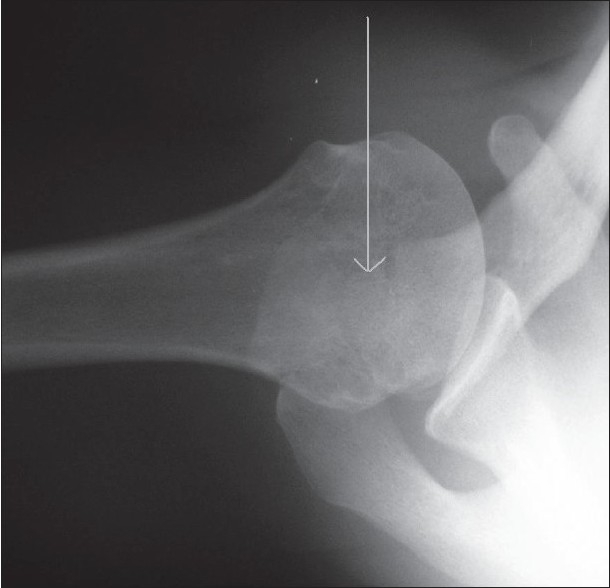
Preoperative axillary shoulder radiograph; the arrow is pointing to the os acromiale
